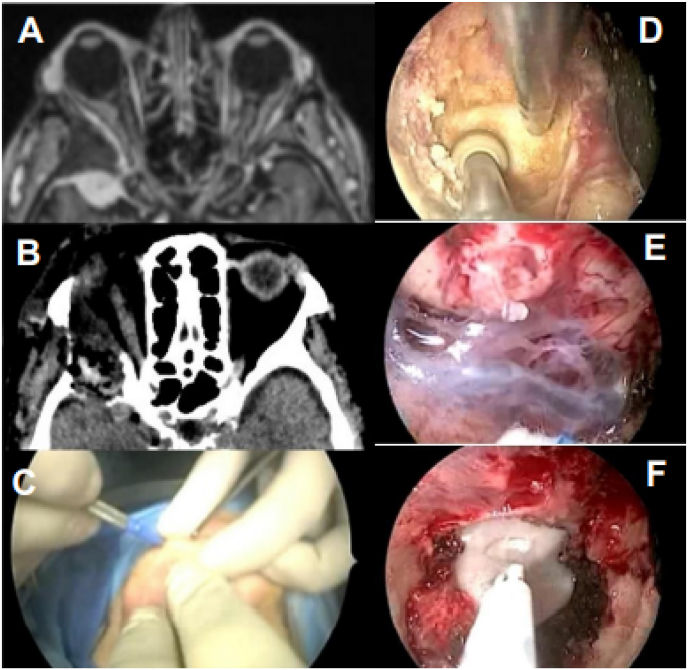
(A) Preoperative MRI showing the presence of temporopolar meningioma with involvement of the sphenoid and of the lateral wall of the orbit (B) postoperative CT showing the orbital decompression and removal of the lesion (C) right upper eyelid incision (D, E) intraoperative endoscopic views (F) dural plastic.
